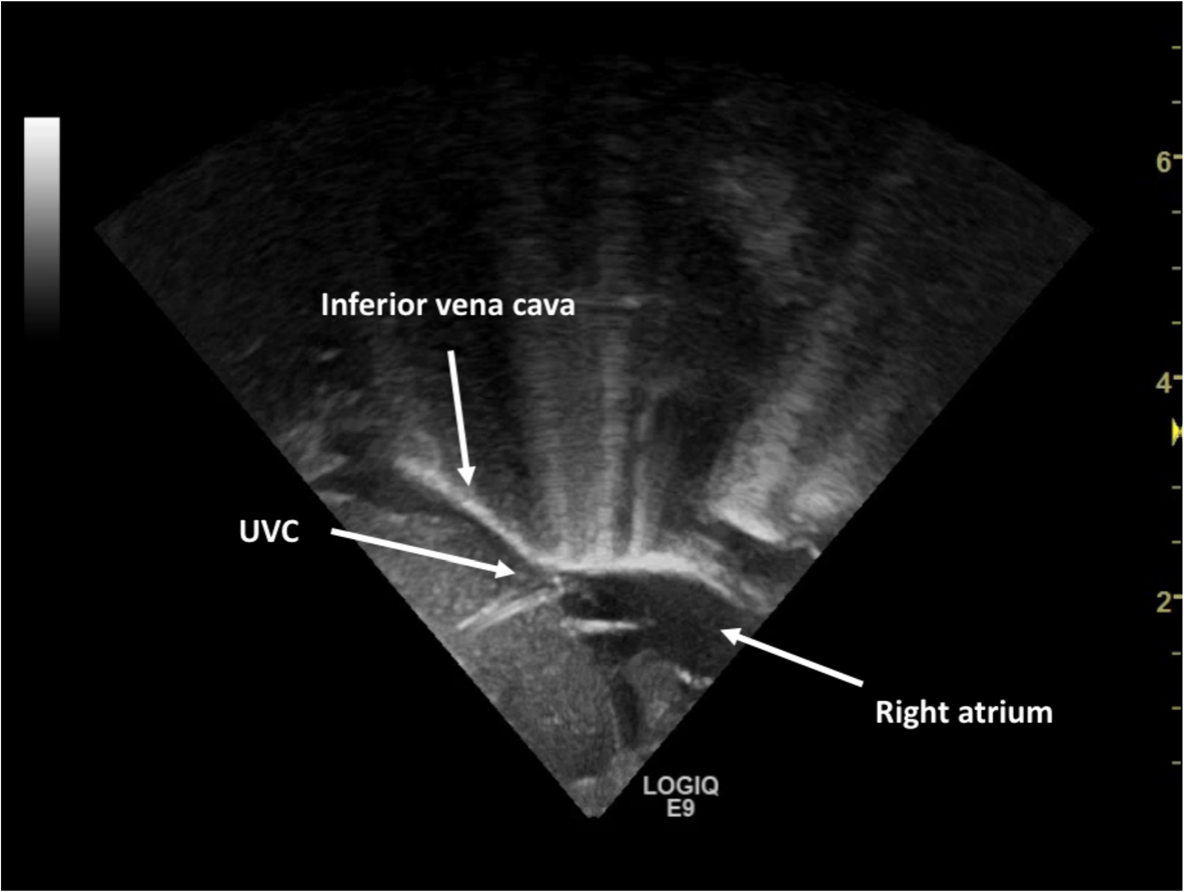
Ultrasound scan of the correct UVC tip position at the junction of inferior vena cava-right atrium
